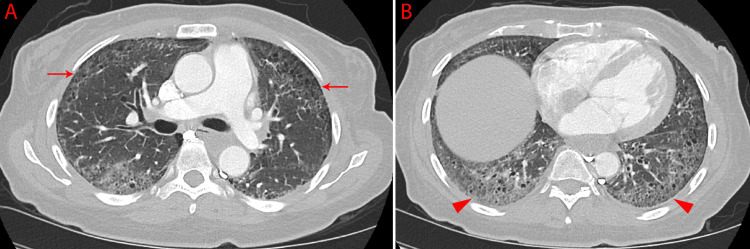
A CT scan of the chest, axial view—A) bilateral peripheral traction bronchiectasis (arrows); B) bilateral peripheral traction bronchiectasis with extensive ground-glass opacification (arrow heads)
